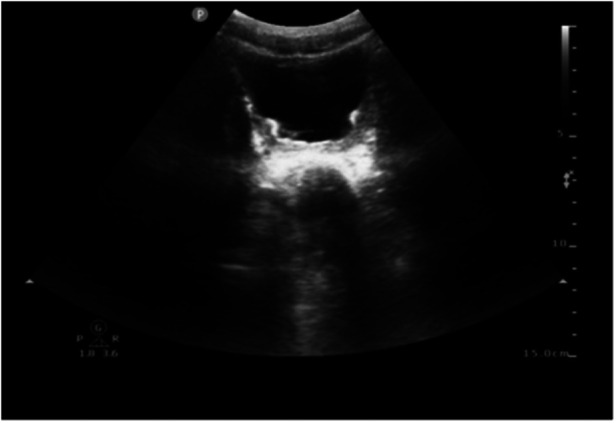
Ultrasound image of bilateral kidneys/ureters/bladder. Ultrasound revealed insufficient bladder filling and an irregular thickening of the posterior bladder wall measuring up to 10 mm.
